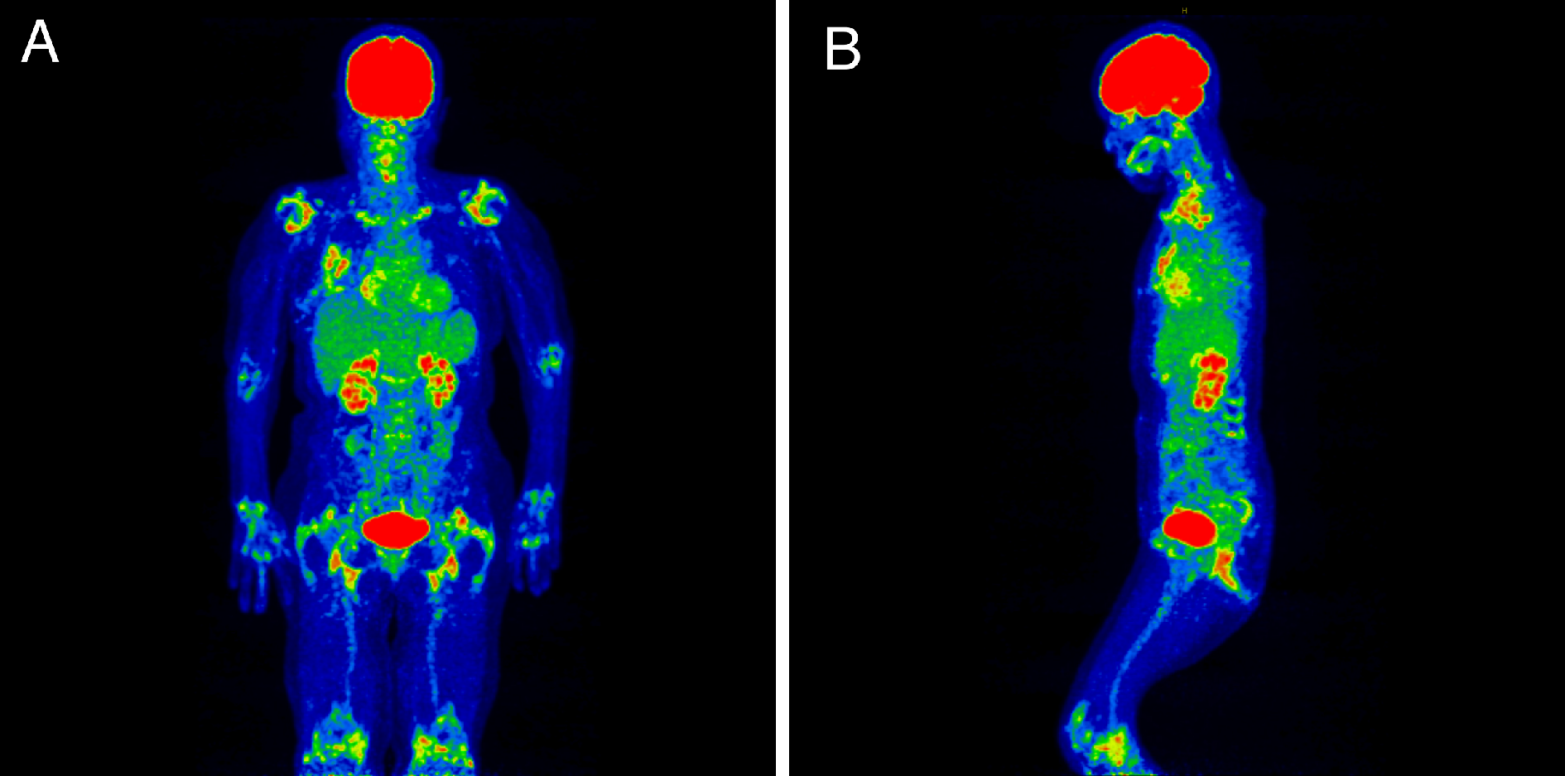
(A) 18-fluorine-fluorodeoxyglucose positron emission tomography/computed tomography (^18^F-FDG-PET/CT) of a 76-year-old woman, showing FDG uptake in bilateral shoulders, sternoclavicular joints, elbows, wrists, hip joints, greater trochanters, ischial tuberosities, and knee joints. FDG accumulation was also observed in the surgical wound on the right anterior chest after an aortic valve replacement. (B) ^18^F-FDG-PET/CT showed FDG uptake in lumbar spinous processes and hip joints.
